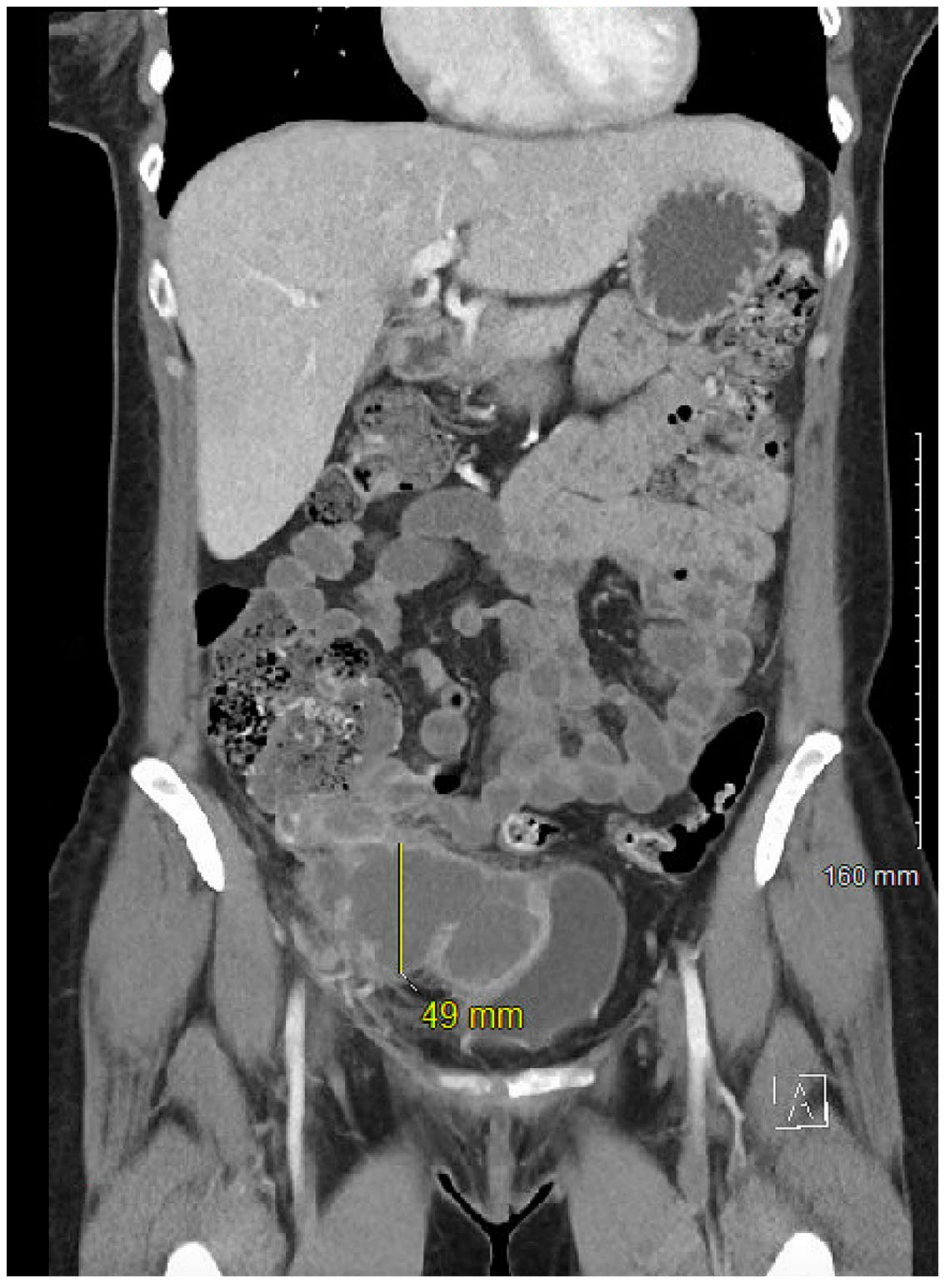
CT image of appendiceal adenocarcinoma.
